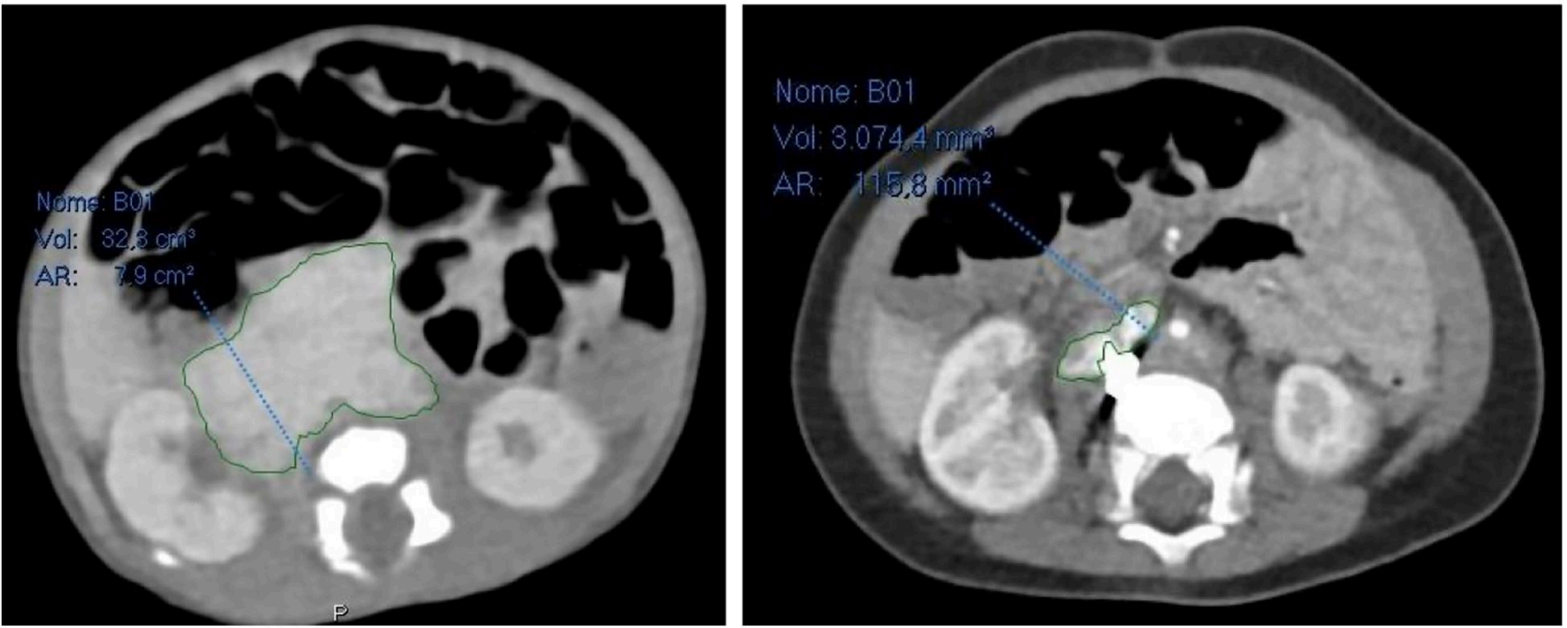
On the left abdominal CT scan at admission, on the right abdominal CT scan 6 months after embolization.
